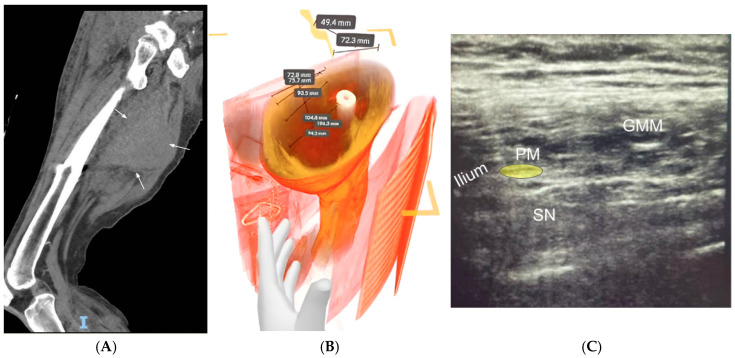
Patient 6, who underwent an ultrasound-guided sciatic nerve block for sciatic neuralgia caused by a large sarcoma involving the adductor musculature. (A) CT scan demonstrated the involvement of most of the pathway of the sciatic nerve by the tumor (white arrows). (B) Virtual three-dimensional reconstruction of the CT scan. The mass was measured in multiple dimensions, taking special note of the cephalad extent of the tumor. A modified subgluteal sciatic approach, closer to the greater sciatic foramen, was planned. (C) Ultrasound-guided block of the sciatic nerve deep to piriformis, safely above the superior margin of the tumor. GMM = gluteus maximus muscle, PM = piriformis muscle, SN = sciatic nerve.
